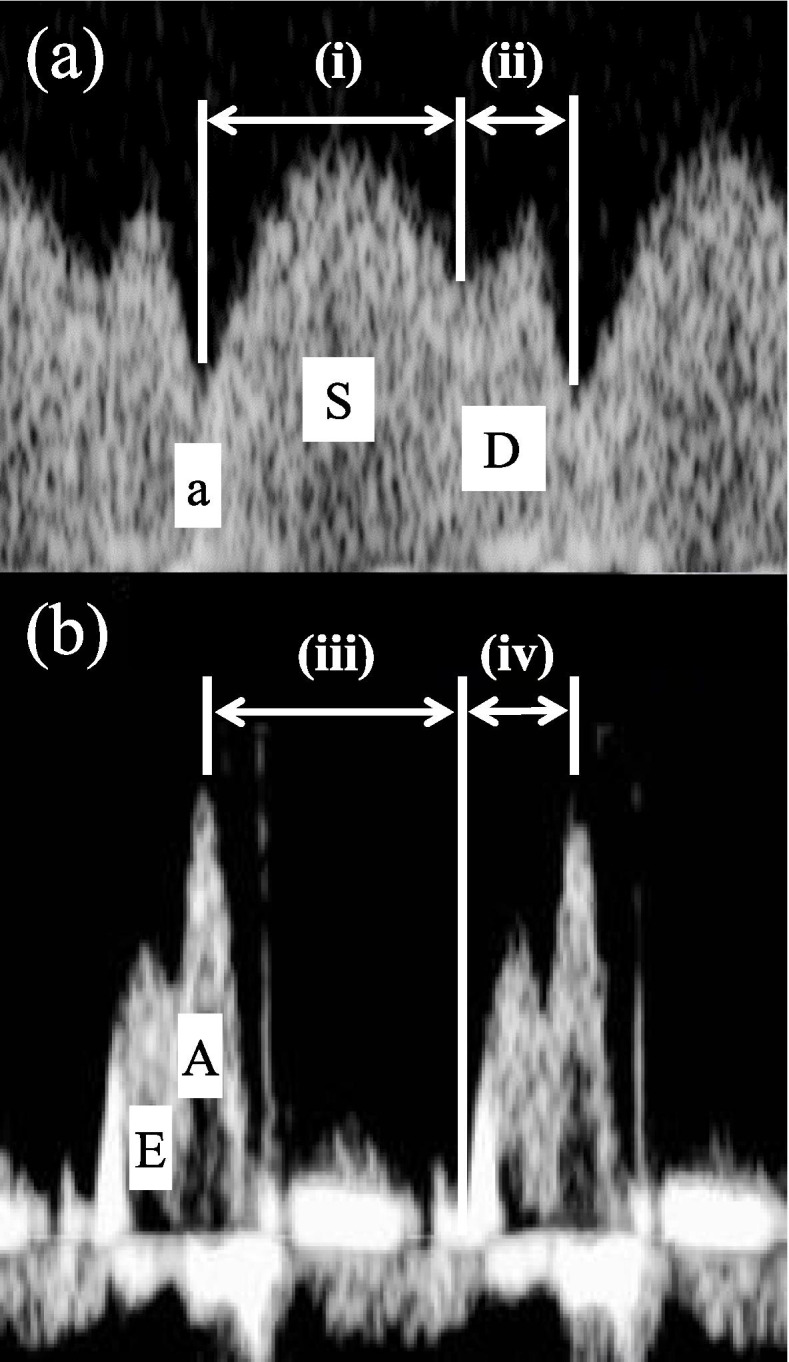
Doppler tracings of flow velocity waveforms (FVW) of ductus venosus (DV) (a) and ventricular inflow (b). (i) indicates time interval for S-wave of DV-FVW, measured from nadir of a-wave during atrial contraction to nadir between S-wave and D-wave; (ii) indicates time interval for D-wave of DV-FVW, measured from nadir between S-wave and D-wave to nadir of a-wave; (iii) indicates time interval from the second peak of ventricular inflow caused by atrial contraction (A-wave) to the opening of the tricuspid and mitral valves; (iv) indicates time interval from the opening of the tricuspid and mitral valves to the peak of the A-wave. (i)/(ii) expressed as DV-S/D. (iii)/(iv) was expressed as TV-S/D for tricuspid valve, and MV-S/D for mitral valve, respectively
